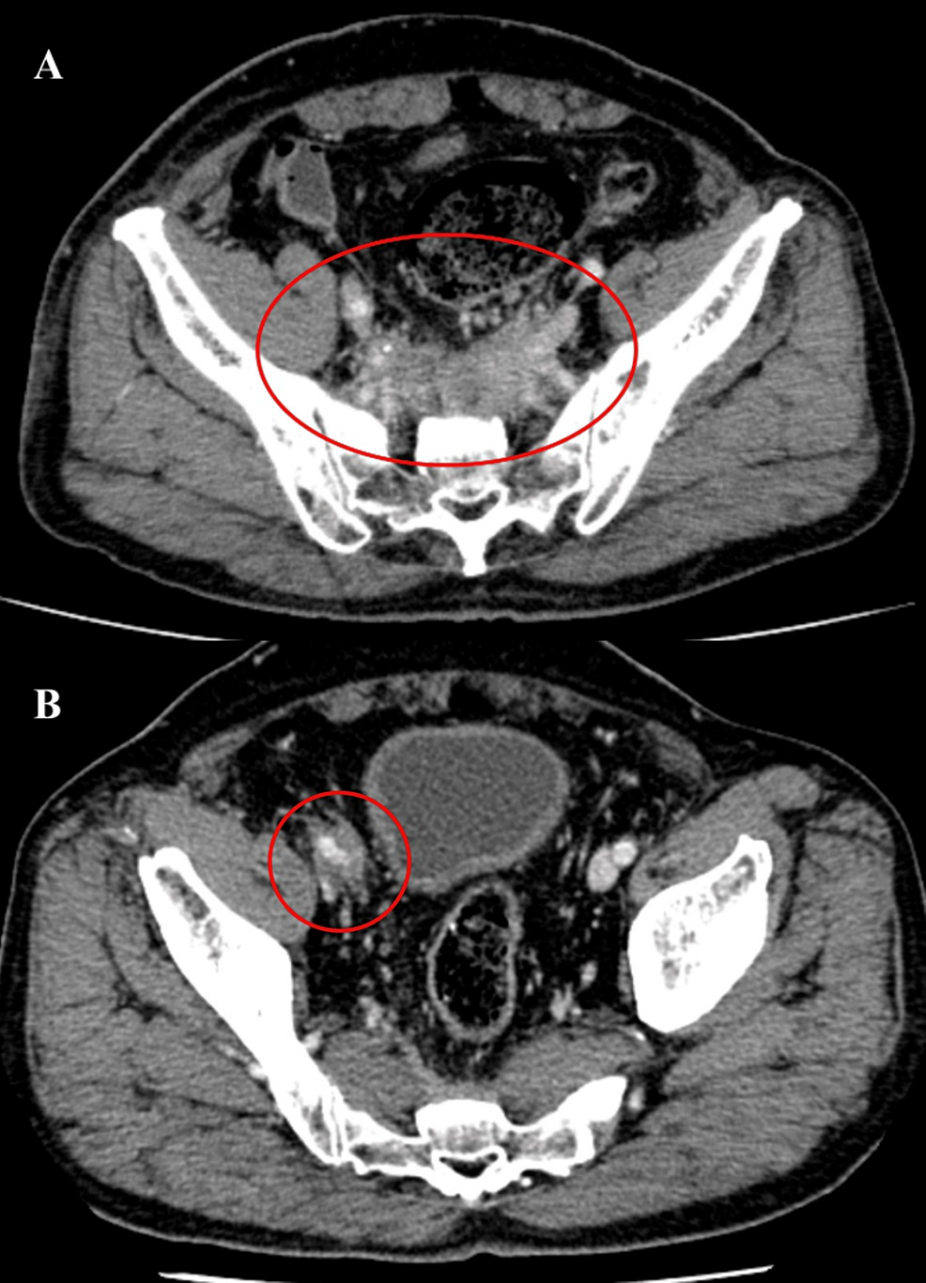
Contrast-enhanced abdominal computed tomography Contrast-enhanced abdominal computed tomography revealed soft-tissue shadows around the ventral sacrum (A) and right external iliac artery and vein (B).
